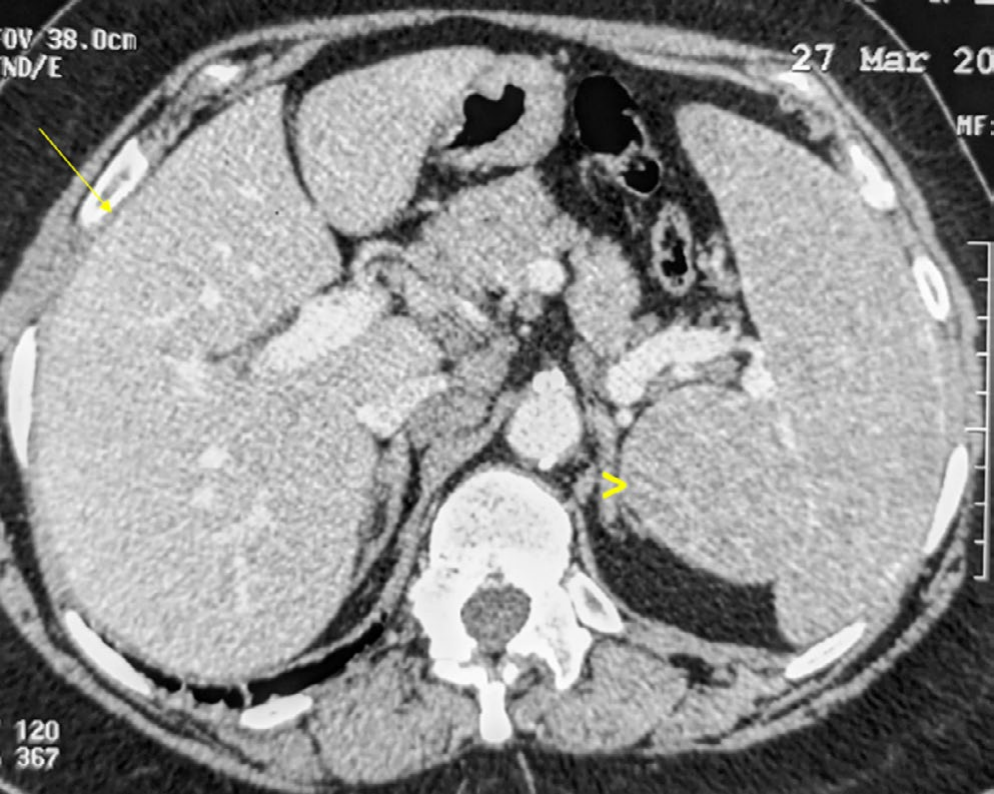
Axial CT of the abdomen shows hepatomegaly (arrow) and splenomegaly (arrowhead)
